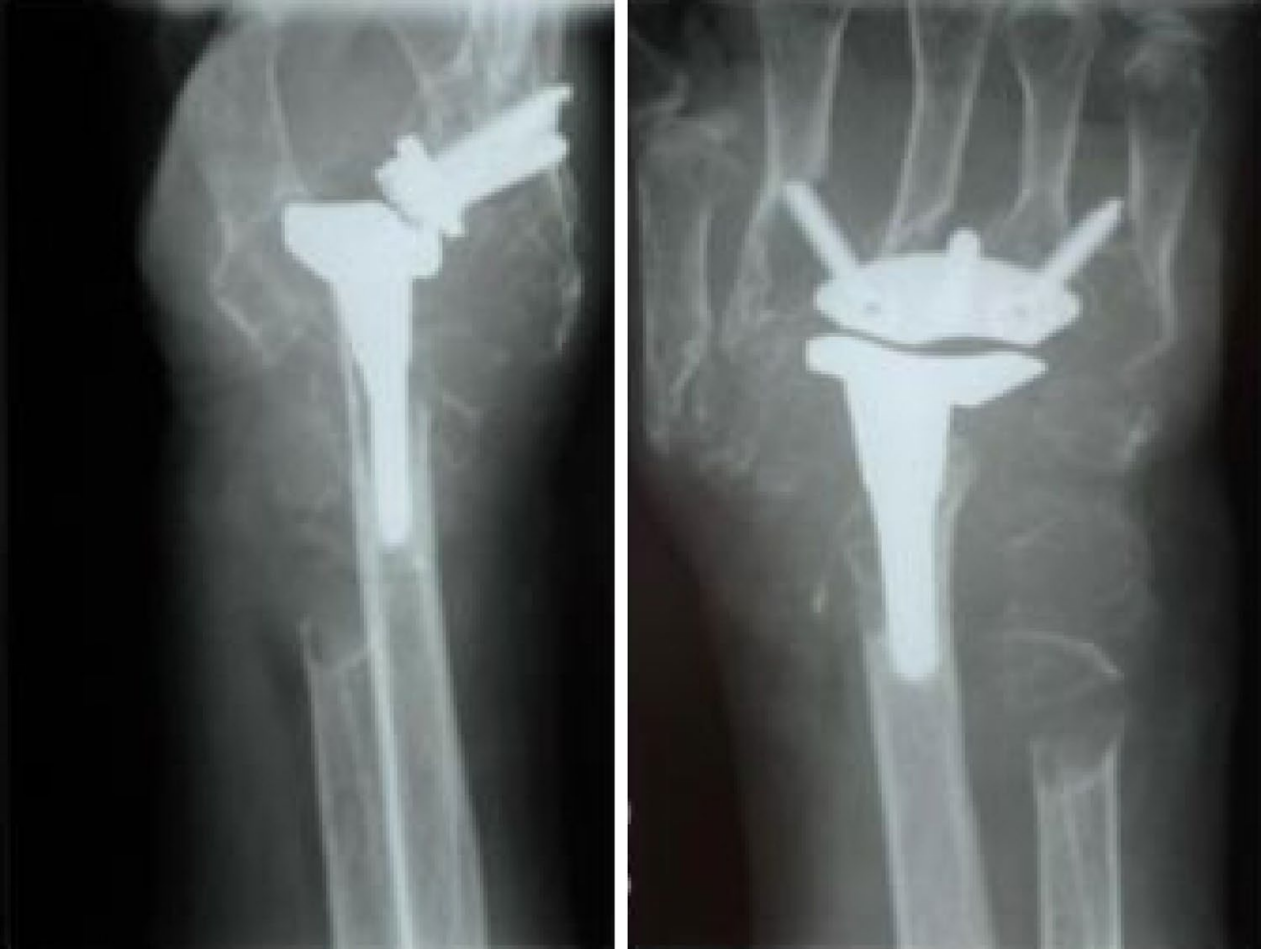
Case 3– Postoperative X-ray of the right wrist (lateral and a.p. view) 16.4 years after revision surgery, replacing the BIAX prosthesis with a Universal II prosthesis
